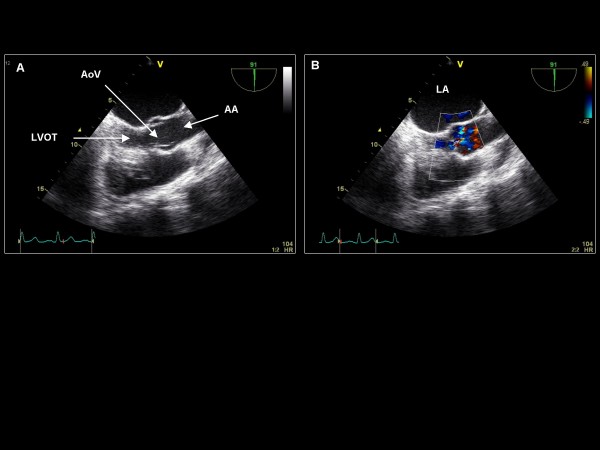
Imaging plane 90°, (A) The LVOT, aortic valve (AoV), and ascending aorta (AA). (B) Color Doppler is applied to the LVOT and aortic valve.
